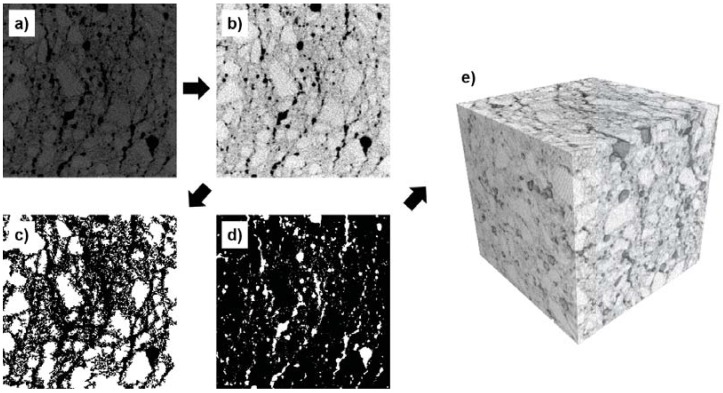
μ-CT imaging process to investigate pore and solid characteristics: (a) original μ-CT image; (b) contrasted image; (c) binarized image for aggregates; (d) binarized image for pores; (e) 3D μ-CT image (Note: in (c) and (d), the white regions represent aggregates (c) and pores (d) while the black is a background.).
